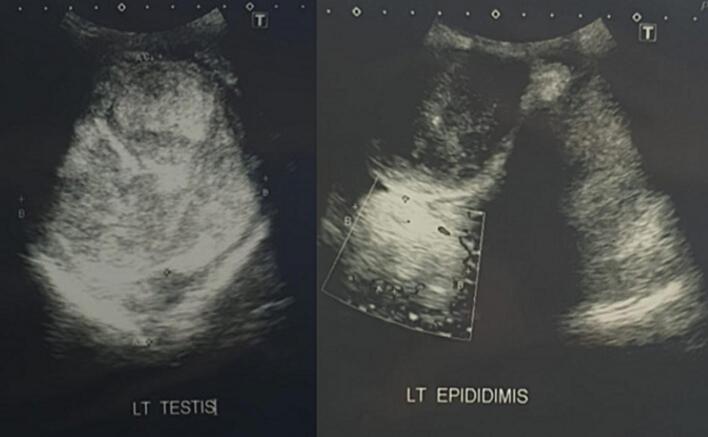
Testicular ultrasonography with doppler.
